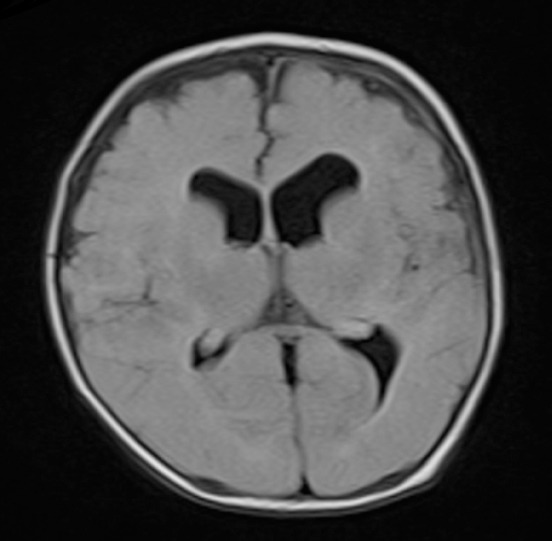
T2-Dark fluid MRI showing left temporal extra-axial collection (10 mm) in a newborn
